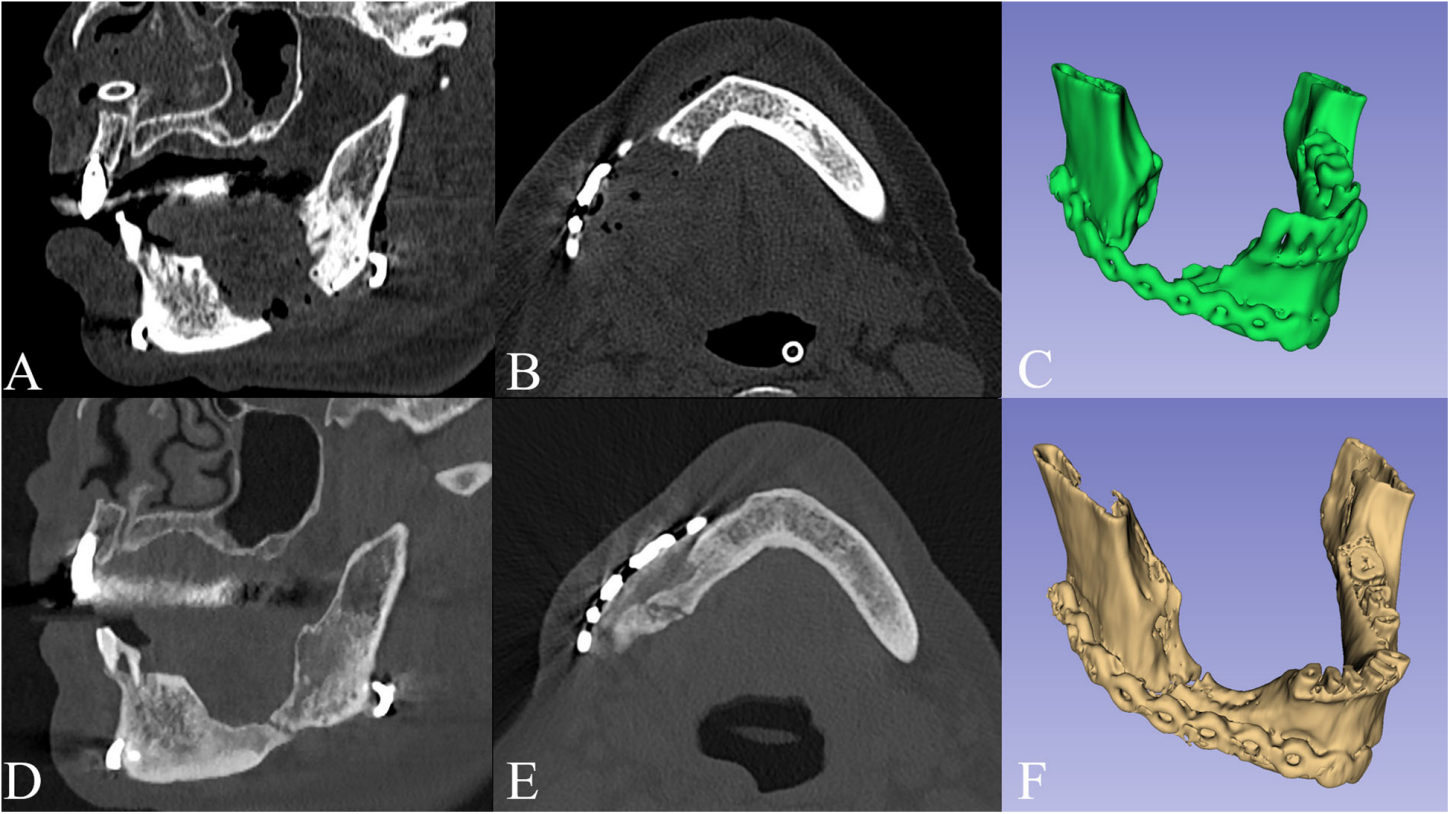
Sagittal view (A), axial view (B) and 3D model (C) of the postoperative CT scan, compared to the 2 years follow-up (D–F), showing bone regeneration at the lower mandibular margin within the surgical gap.
